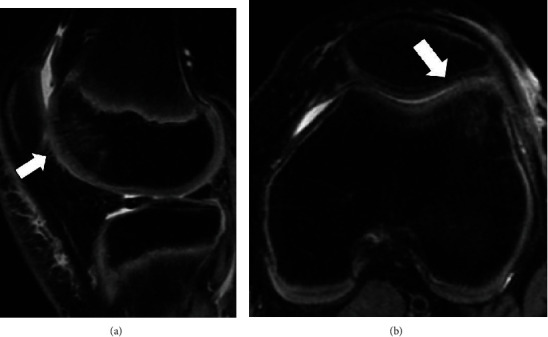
Postoperative sagittal (a) and axial (b) T2-weighted, fat-suppressed magnetic resonance images taken 2 months after osteochondral peg fixation showing a complete bony and chondral union and a continuous articular surface.
